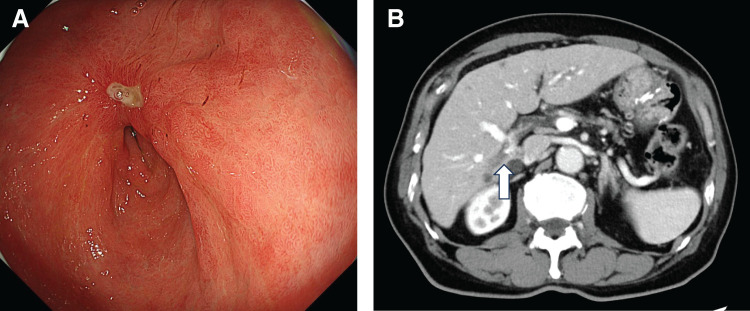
Post-chemotherapy imaging findings. (A) Upper gastrointestinal endoscopy showed marked regression of the gastric lesion. (B) Contrast-enhanced CT revealed that the liver metastasis in S6 had decreased in size to 2.1 cm (white arrow).
